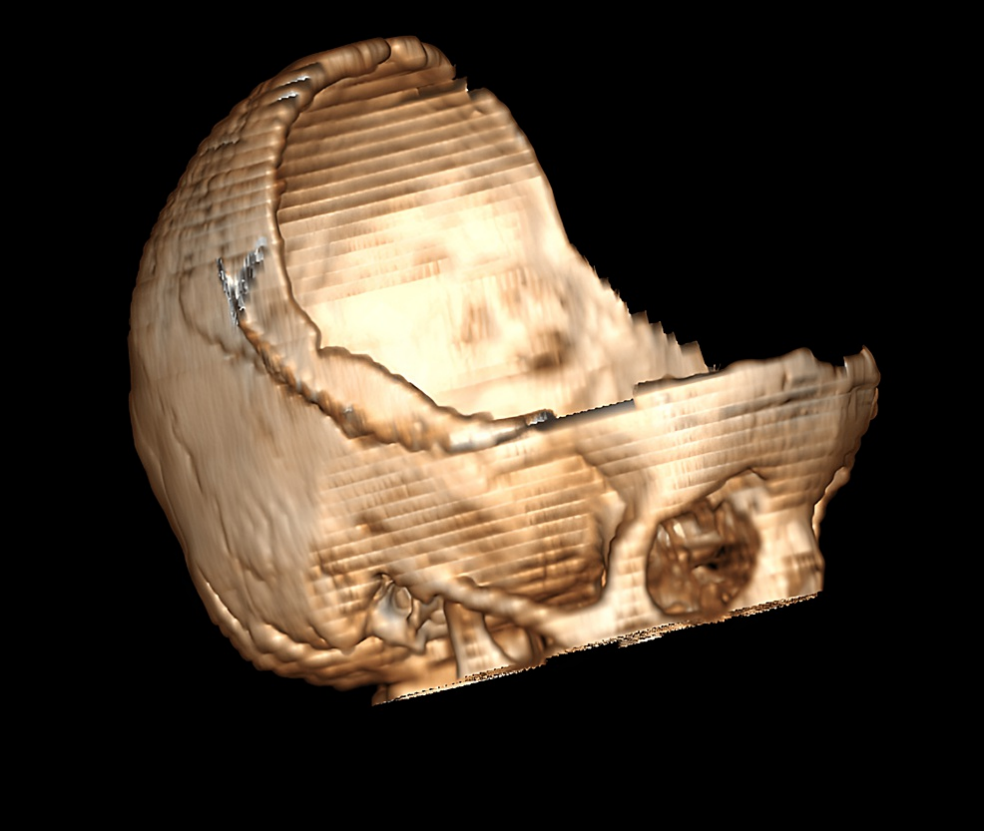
3D animation of postoperative brain CT.
